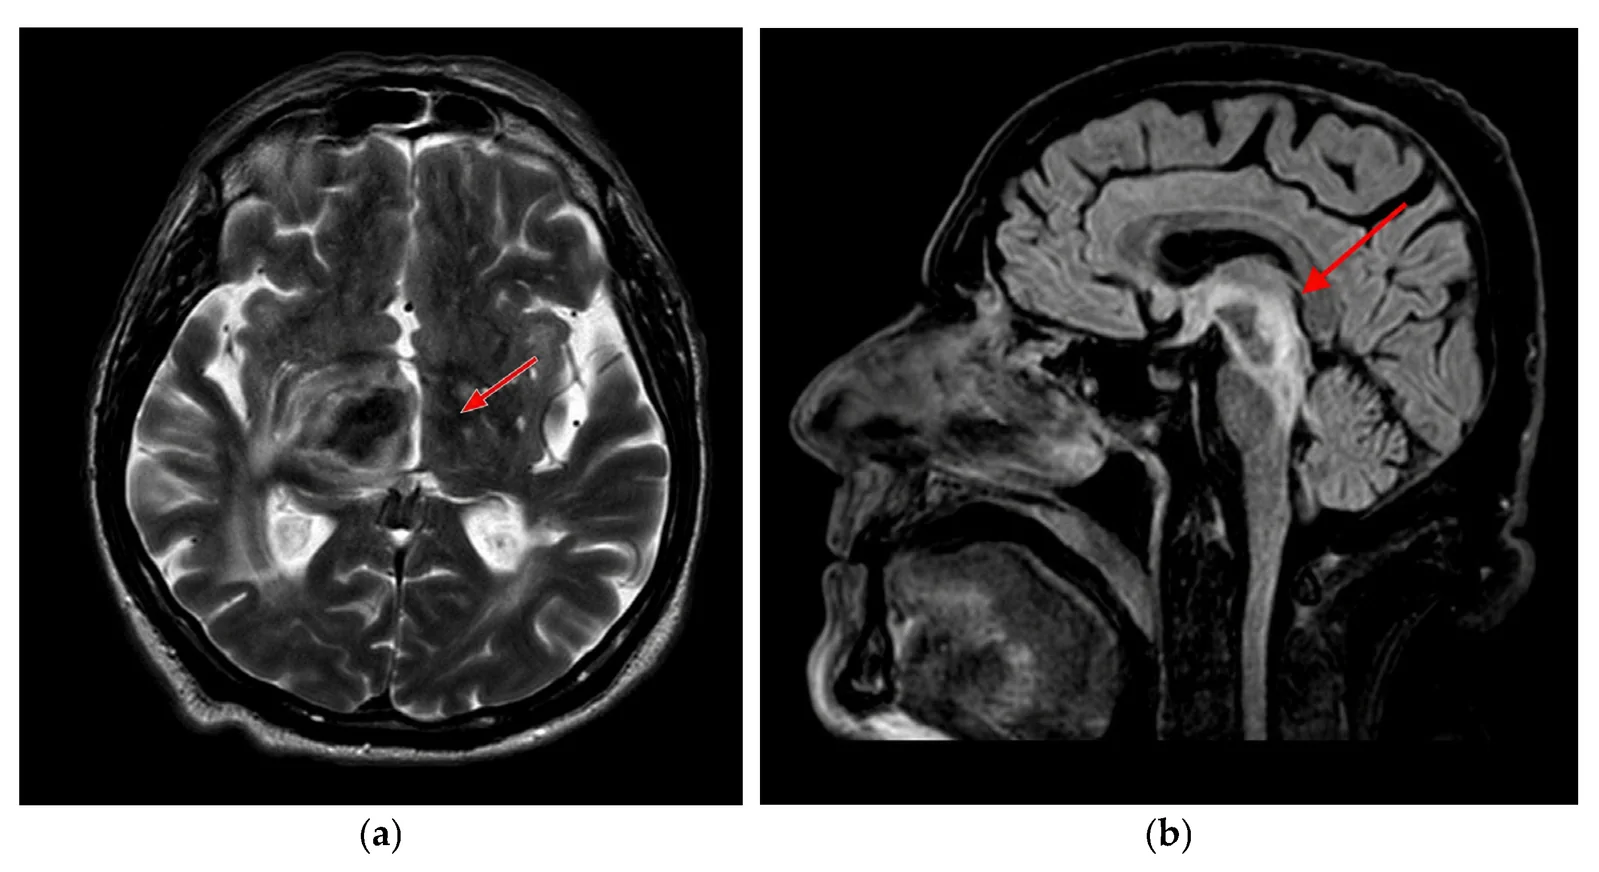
Representative brain MRI images: (a) Axial T2-weighted image showing a right thalamo-mesencephalic lesion with heterogeneous signal intensity and surrounding edema. (b) Sagittal FLAIR image showing the cranio-caudal extension of the lesion and its involvement of the diencephalic–mesencephalic region. Red arrows indicate the right thalamo-mesencephalic hemorrhagic lesion.
